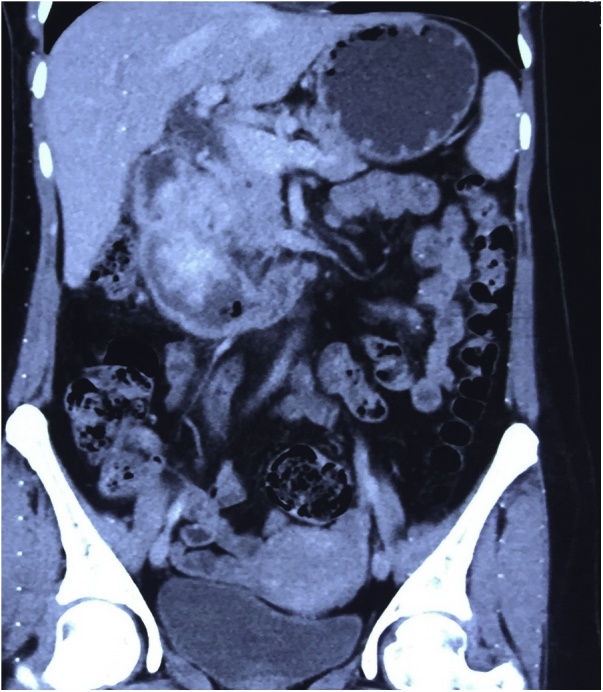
CT-scan of the abdomen showing an irregular mass with mixed enhancement arising from the region of the head of the pancreas.
